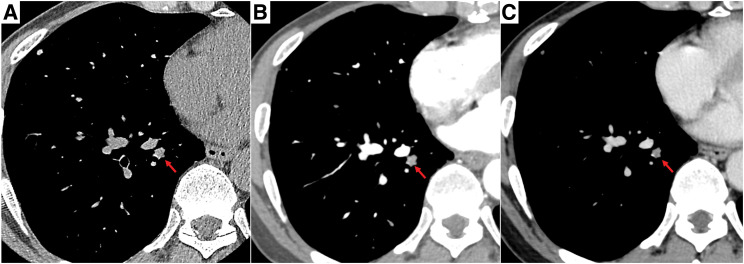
Plain CT (A), early-phase contrast-enhanced CT (B), and delayed-phase contrast-enhanced CT (C) are shown. The pulmonary nodules exhibited nonspecific mild enhancement, and peripheral microcalcifications were observed in approximately one-third of the nodules (representative lesions are indicated by red arrows).
